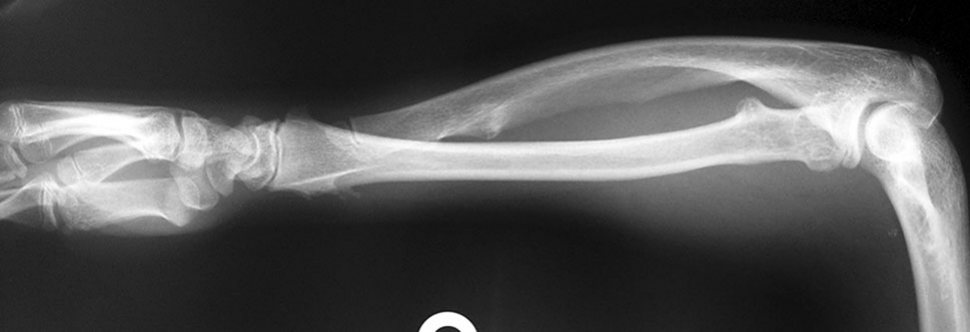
A fourteen-year-old boy with Congenital Multiple Exostosis Rt. Ulna treated initially by excision. X-ray showing type 1 deformity in which there is ulnar deviation of the hand and deformity of the radius
